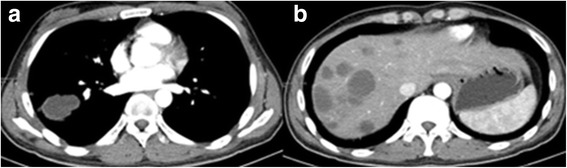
a (left): CT showing the decrease in size of the lung lesions in comparison with Fig. 15a. b (right): CT showing the increase in size of the liver lesions in comparison with Fig. 17
